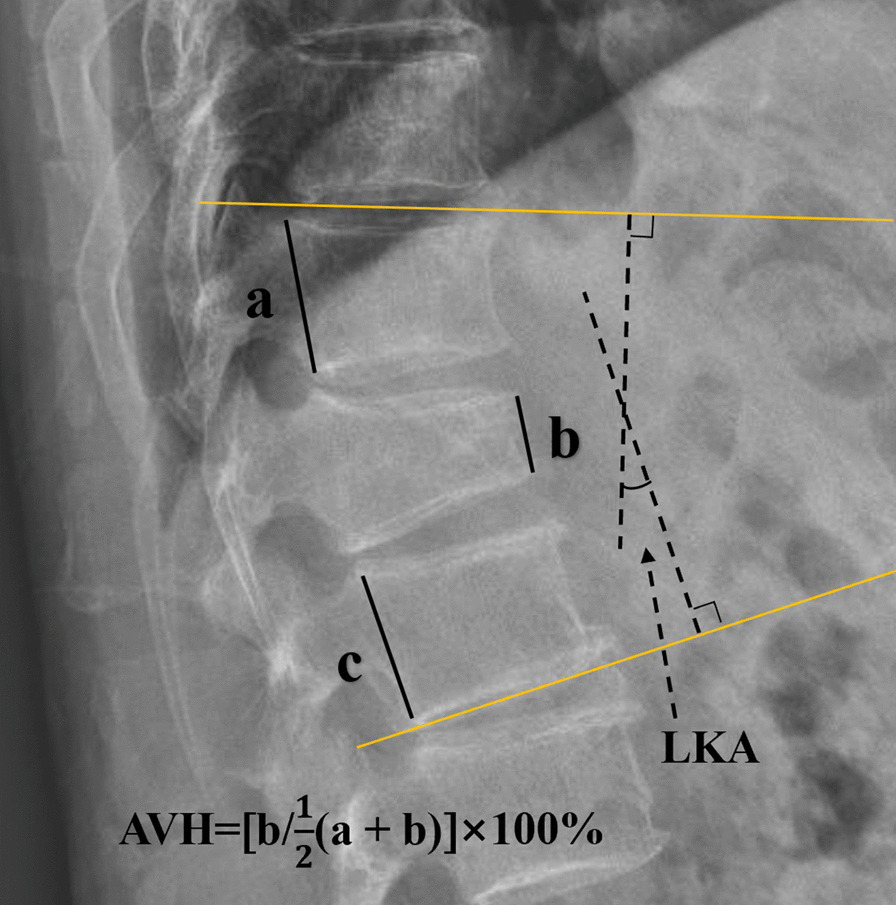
Radiographic evaluation of compressed vertebrae. a Posterior height of the upper vertebrae; b anterior height of the vertebrae; c posterior height of the lower vertebrae; LKA: local kyphotic Cobb’s angle
